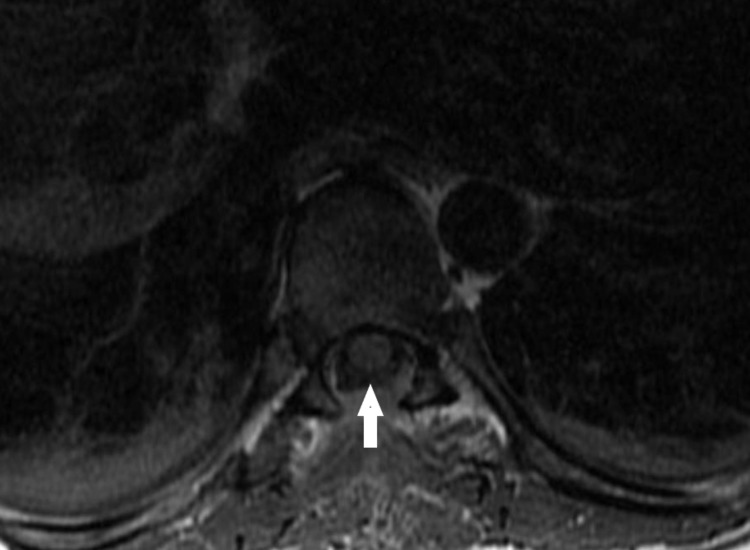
Post-operative axial T1 magnetic resonance imaging (MRI) showing the transverse dimension of the tiny dorsal column lesion in the midline at the T7 level of the thoracic spinal cord The linear hyperintensity above the arrow measures approximately 2 x 5 mm.
